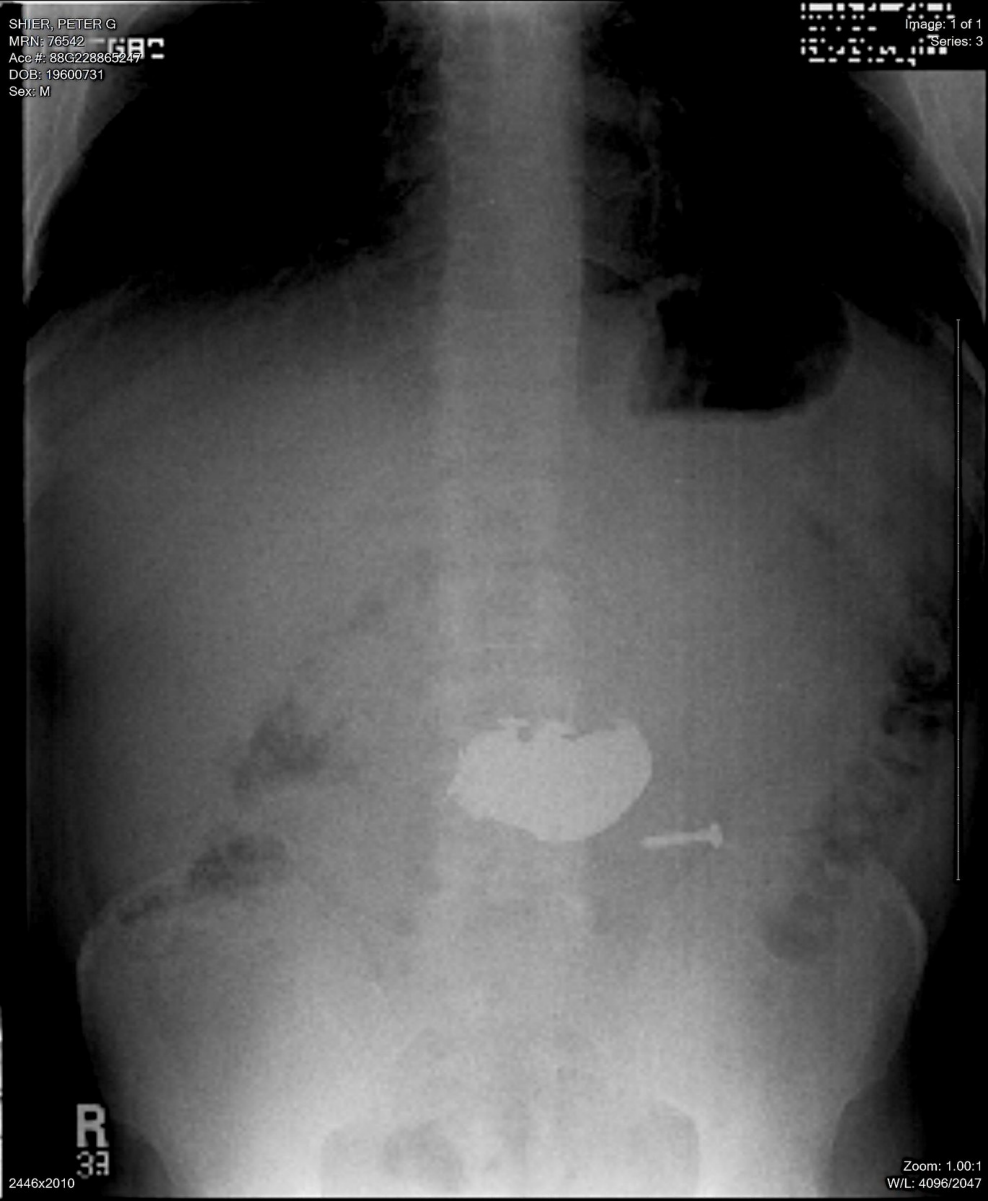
Upright abdominal x-ray demonstrating multiple ingested metallic objects.
